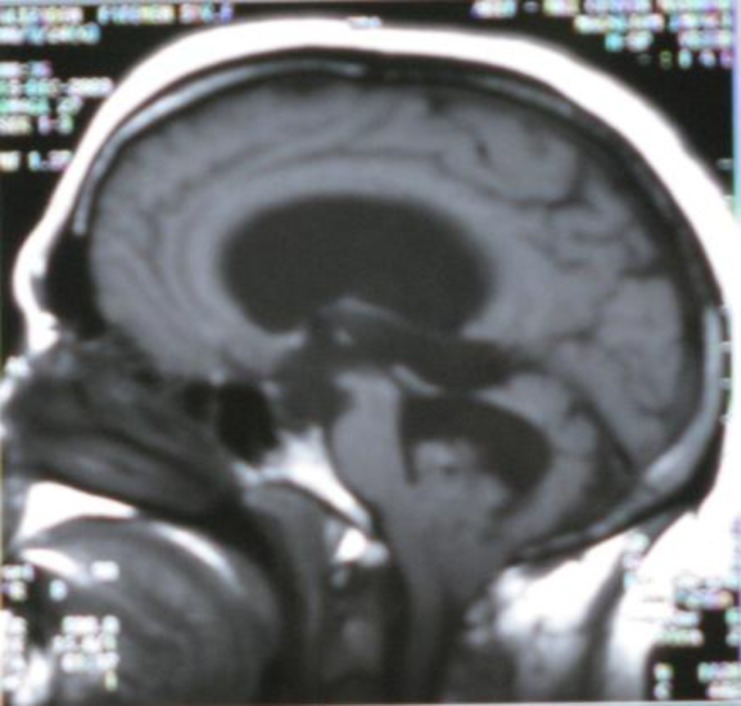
(Sagittal T1-weighted MRI shows isointense tumor in 4^th^ ventricle).
